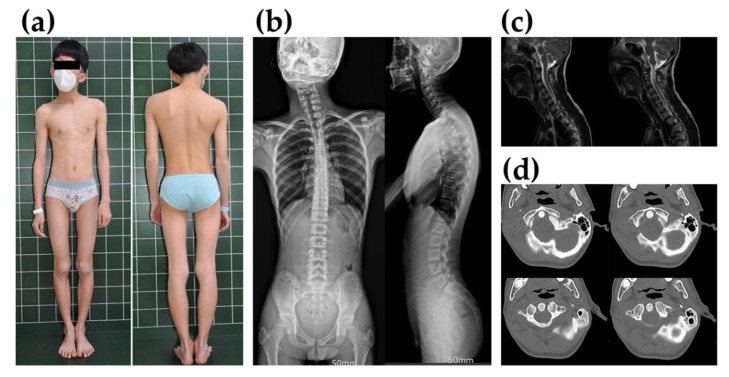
The proband’s phenotype and radiologic findings. (a) The proband with uncorrected muscular torticollis before surgery. His head is tilted toward the left, showing shoulder elevation and facial asymmetry. (b) Simple cervical, thoracic, and lumbar spine radiography. Cervicothoracic curvature was observed due to torticollis. (c) In T2-weighted sagittal magnetic resonance imaging (MRI) of the cervical spine, there were no abnormalities in the spinal cord and cervical disc. (d) Normal atlanto-axial (C1-C2) joint findings on computed tomography (CT).
